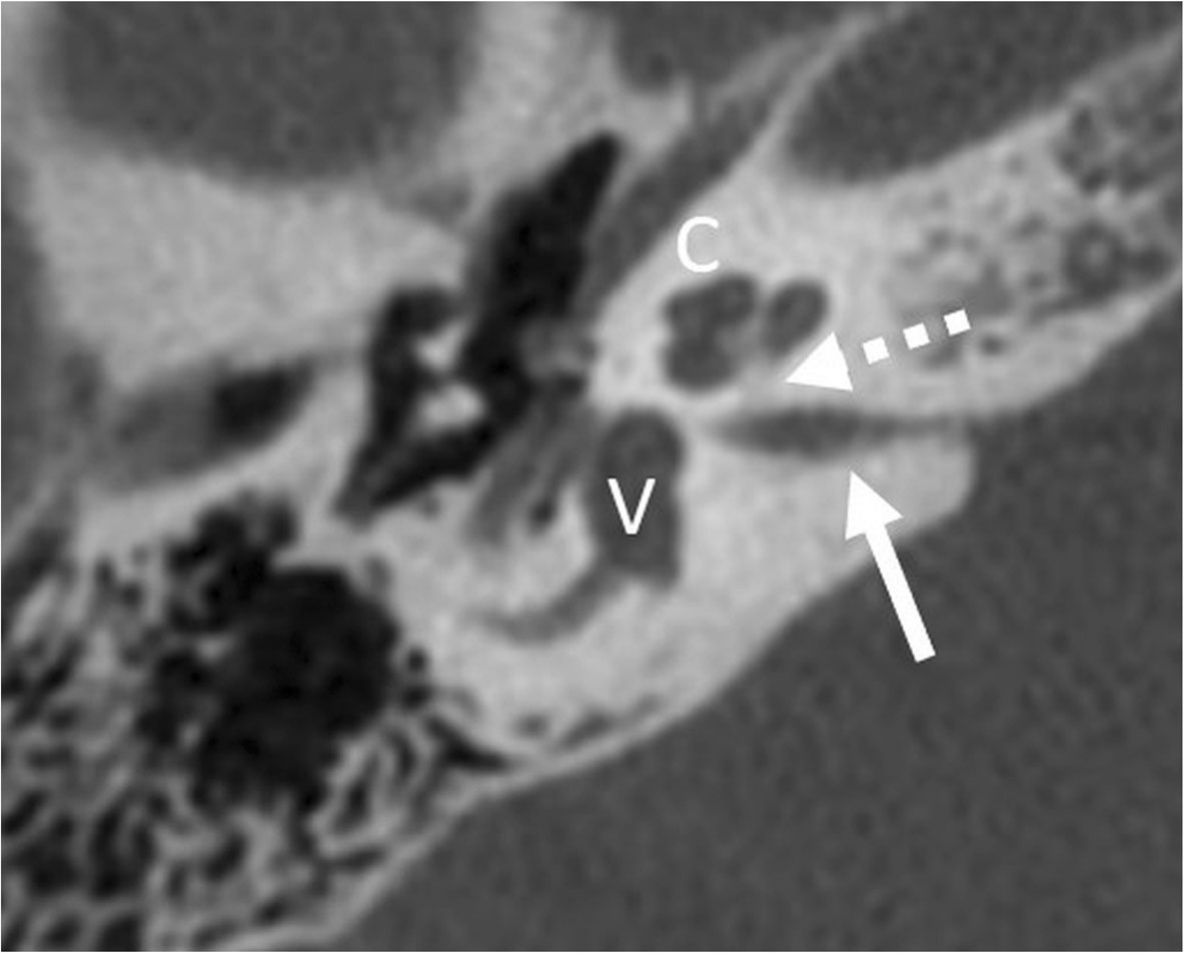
A 16-year-old female patient, with unilateral deafness from birth. HRCT axial image shows high-grade stenotic bony cochlear nerve canal (dashed arrow) and the stenotic internal auditory canal (arrow). Cochlea (C) and vestibulum (V) appear normal
